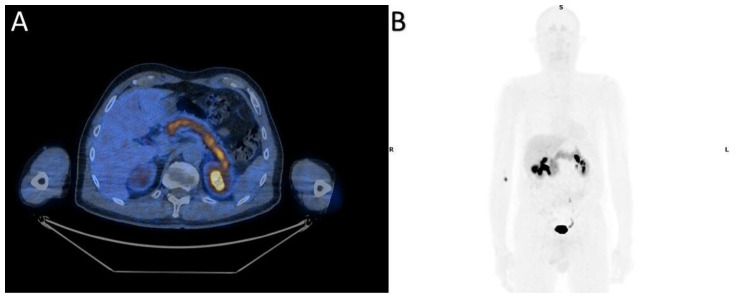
[^18^F]FDOPA PET/CT transaxial images (A) and MIP (B) show diffuse physiological uptake in the pancreas.
